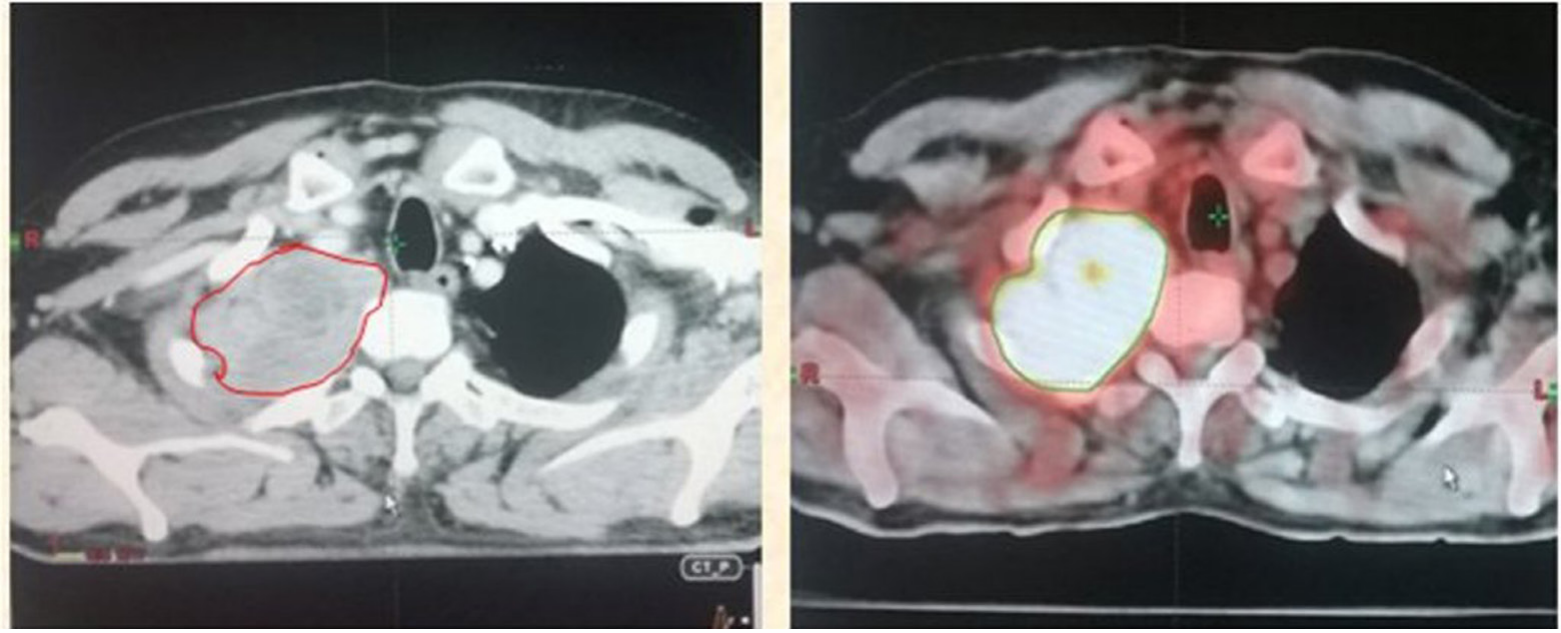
GTV primary tumor volume drawn in red on CECT and green on ^18^F-FDG PET-CT showing increase in GTV volume on ^18^F-FDG PET-CT
